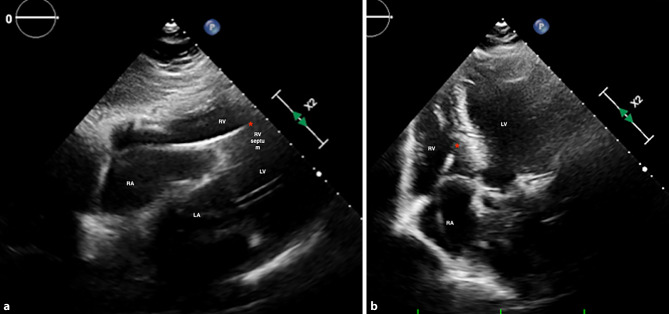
a Subcostal view of the heart with the pacing lead (*) opposed to the right ventricular septum (RV septum). b Apical 4-chamber view of the heart with the pacing lead (*) deployed to the mid-right ventricle septum. *LA* left atrium, *LV* left ventricle, *RA* right atrium, *RV* right ventricle
